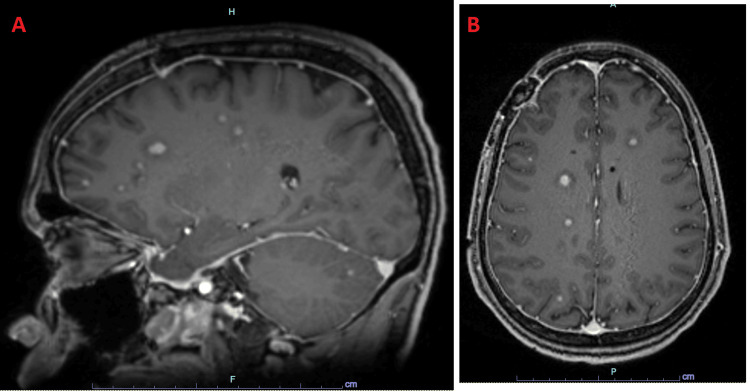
Sagittal and axial MRI three months after presentation. Sagittal (A) and axial (B) MRI three months following the initial presentation demonstrating a marked interval decrease in lesion sizes as well as a reduction in edema (T1 3D space G+ MPR). MRI: magnetic resonance imaging; MPR: multiplanar reformation or reconstruction
